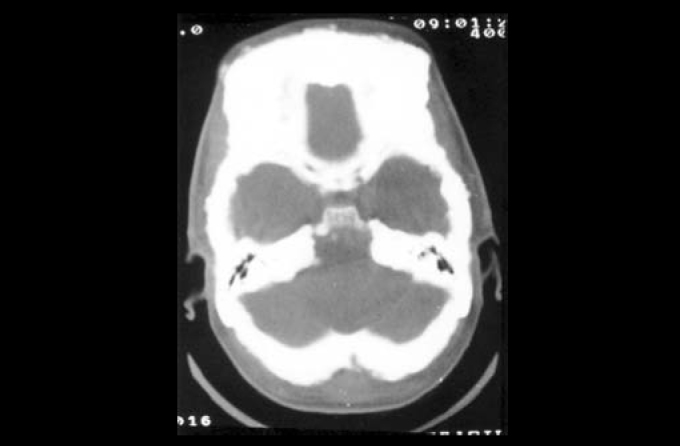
Case 2: CT scan (axial section) showing hyperostotic craniodysplasia, especially in the frontal region, but with diffuse affection.
